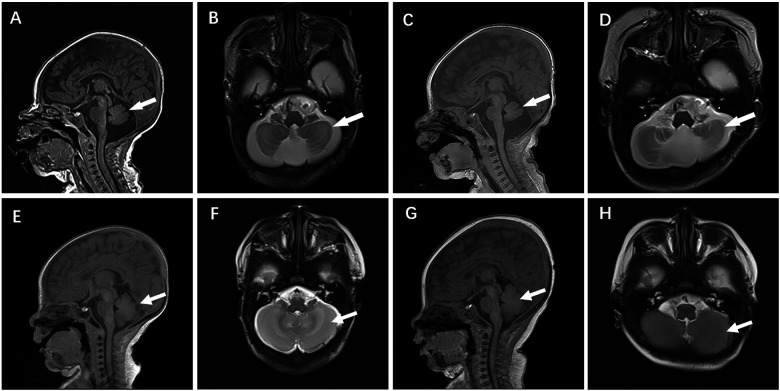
Cranial MR images of case 4 brain MR Images of the 2-week-old showed cerebellar hypoplasia on (A) (T1WI) and (B) (T2WI) images. At 2 months, (C) (T1WI) and (D) (T2WI) images of the same level showed aggravated cerebellar dysplasia. (E–H) displayed a normal skull image of the same age and sex, with the arrows indicating the cerebellum.
